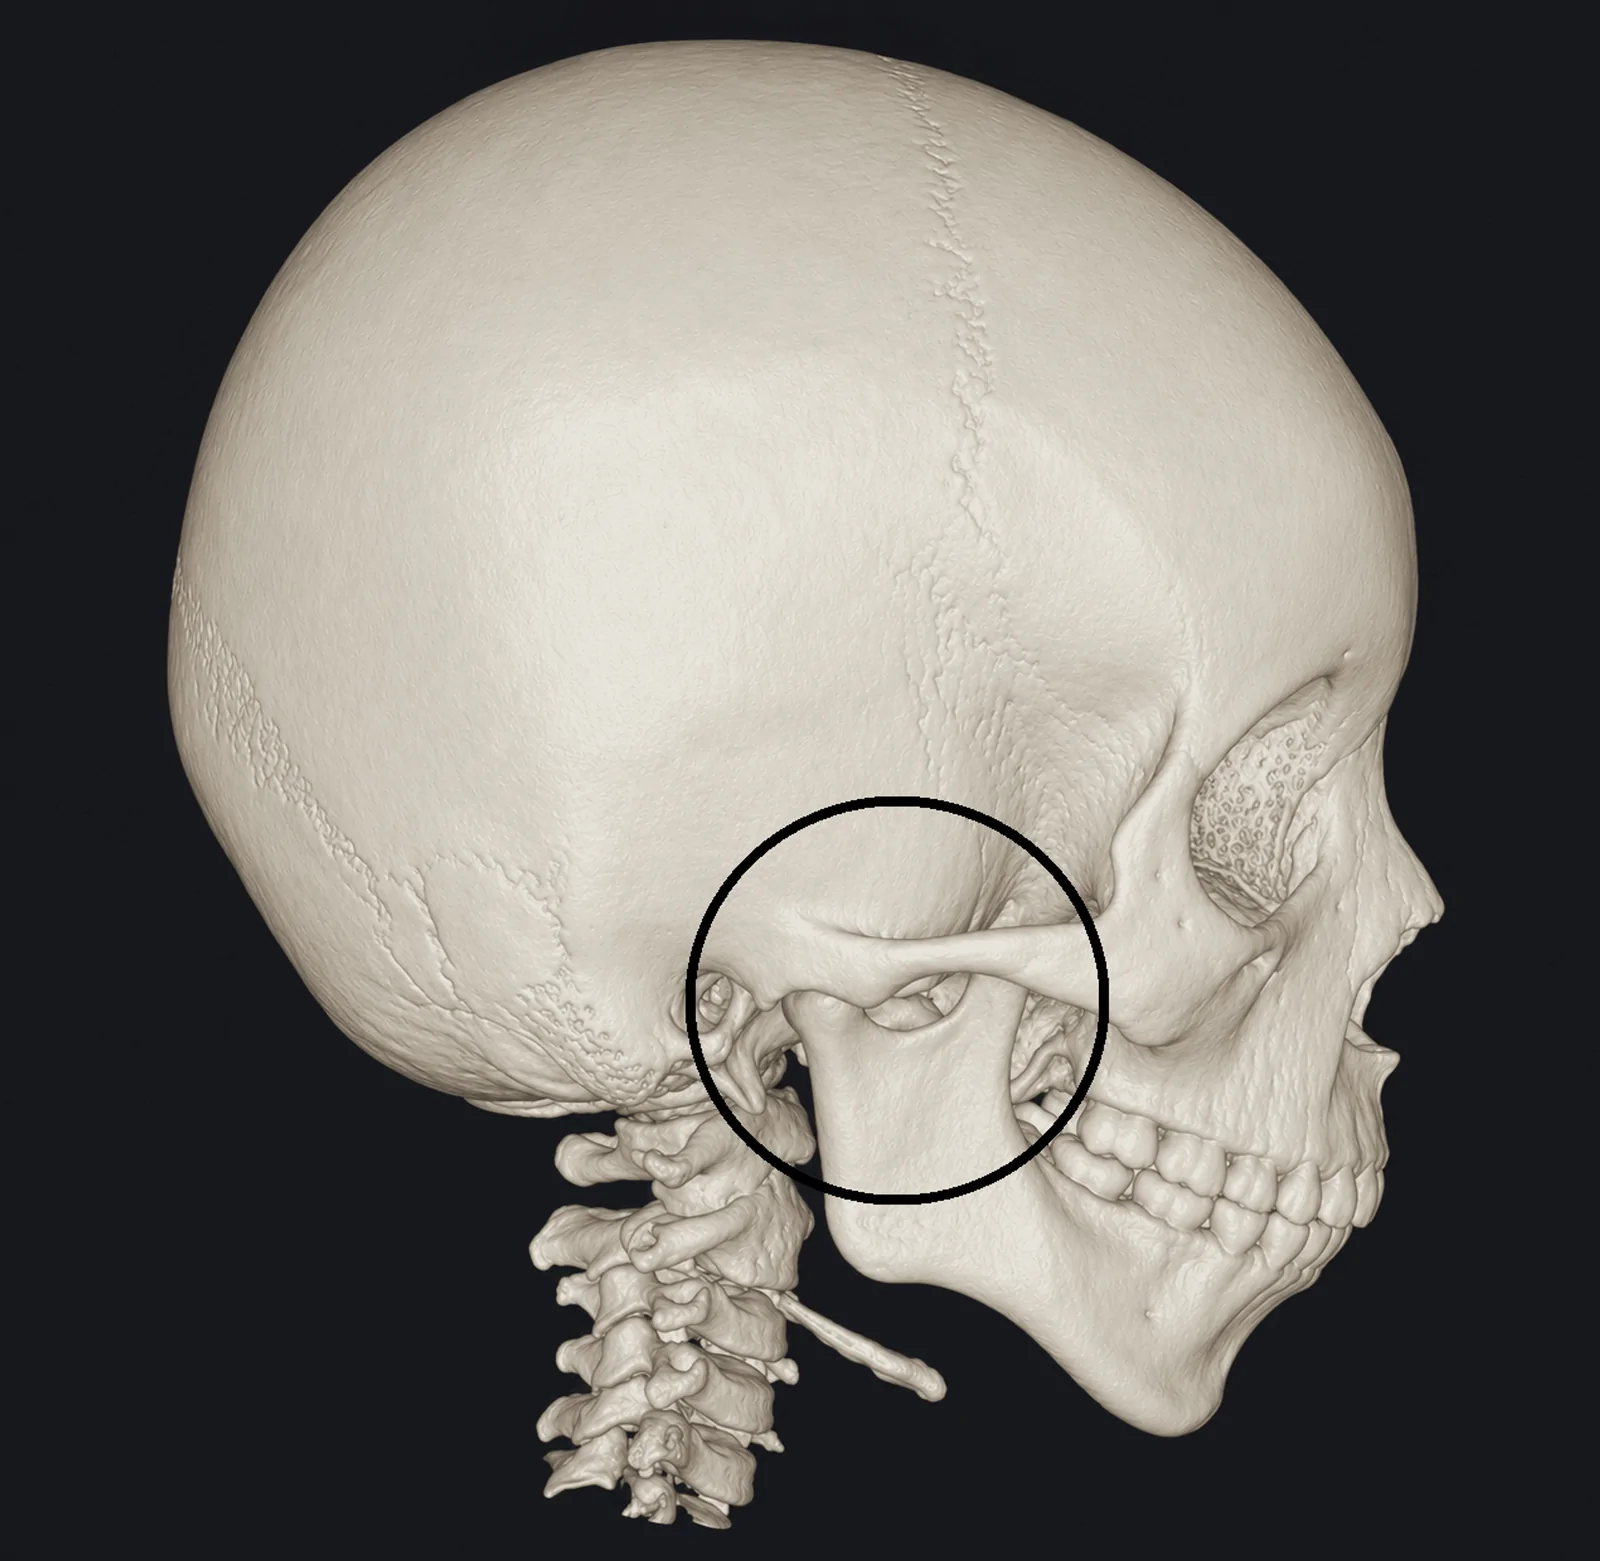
Preoperative three-dimensional computed tomography reconstruction demonstrating right temporomandibular joint bony ankylosis with fusion of the mandibular condyle to the temporal bone, resulting in obliteration of the joint space (black circle).
